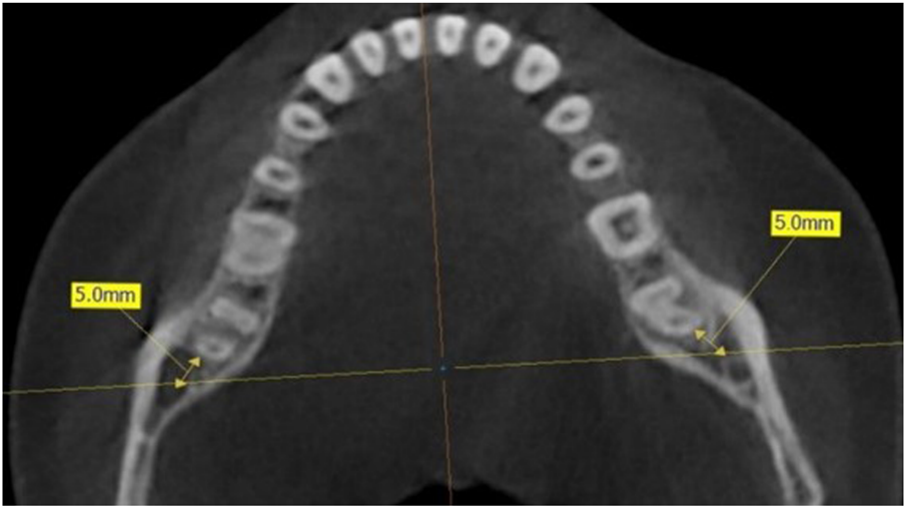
Axial view of the mandible in cone‐beam computed tomogram image with a marked distance of 5 mm distal to the last molar. Coronal view was adjusted using corresponding distance markings.
